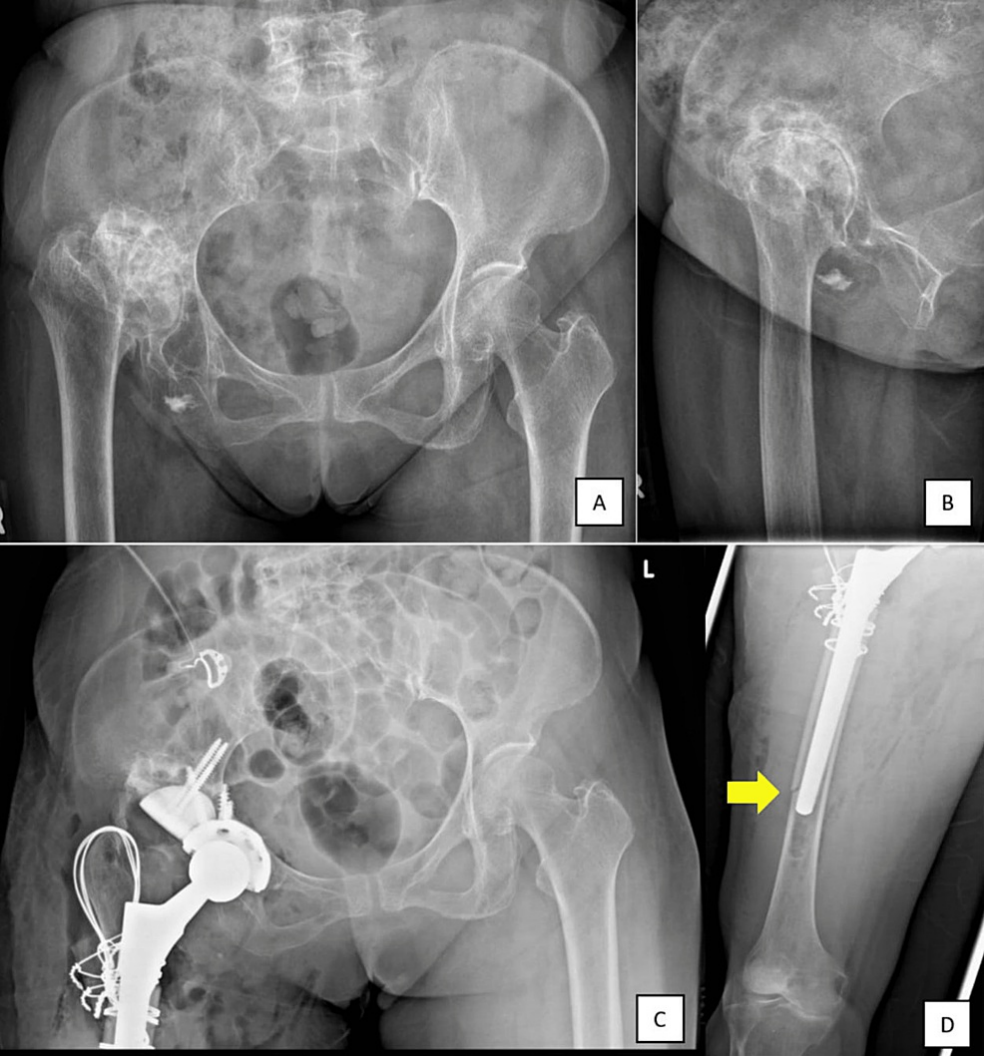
(A) Plain radiograph of the pelvic prior to the surgery; (B) Plain radiograph of the right hip in lateral view; (C) Plain radiograph of the pelvic after the index right total hip replacement (THR). A cementless stem was inserted, and the superolateral dysplastic pseudoacetabulum was reconstructed with acetabulum cup and augmented with trabecular metal; (D) A femur iatrogenic fracture occured during the index total hip reconstruction (yellow arrow).
